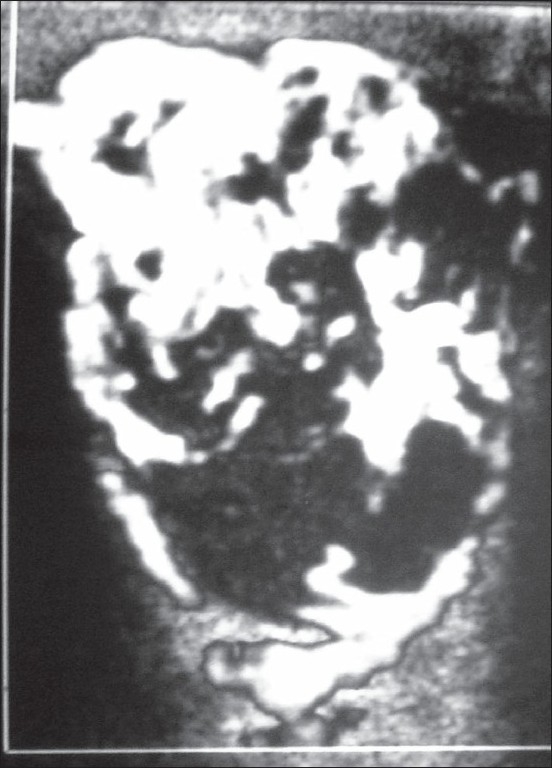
Color Doppler shows an extraconal mass (arrow) with rich vascularity in the superomedial quadrant
